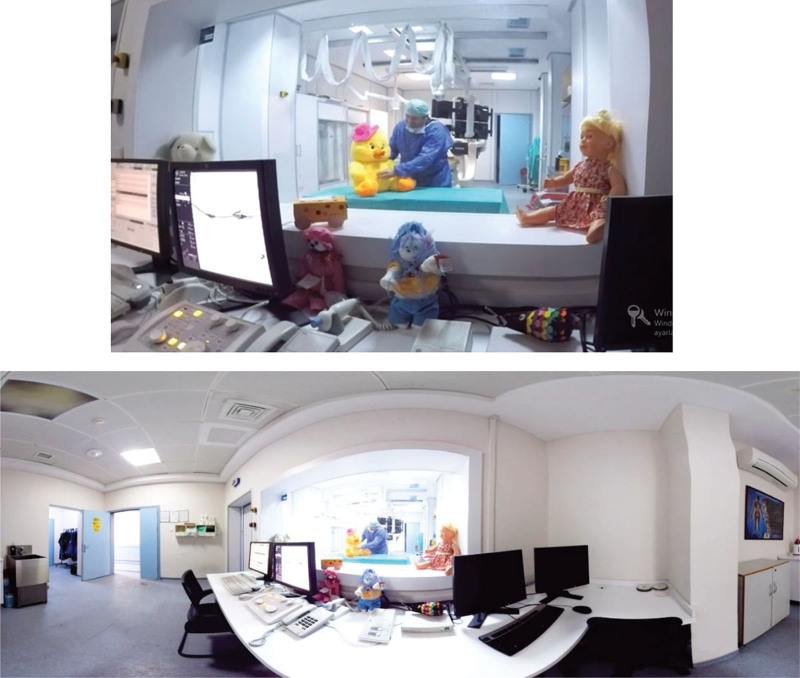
Part of 3D echocardiography film.
